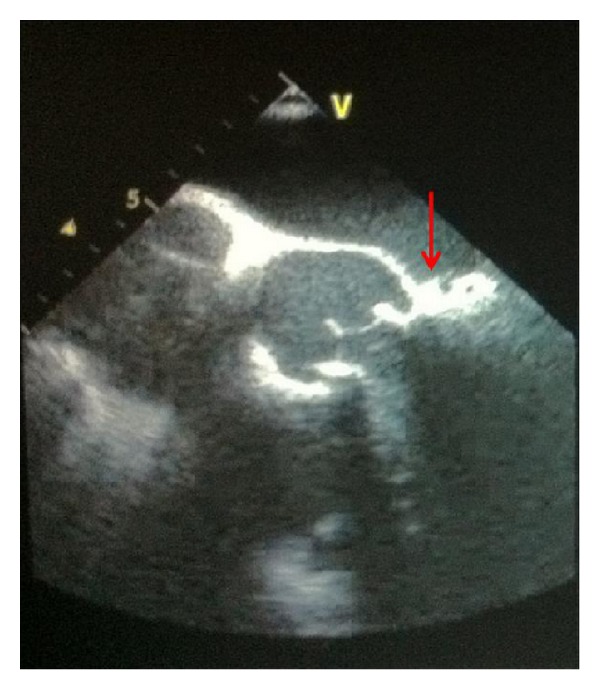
Transesophageal echocardiogram (TEE) showing the aortic valve, mitral valve, and mitral-aortic intervalvular fibrosa (red arrow) with adjacent pseudoaneurysm (P-MAIF).
